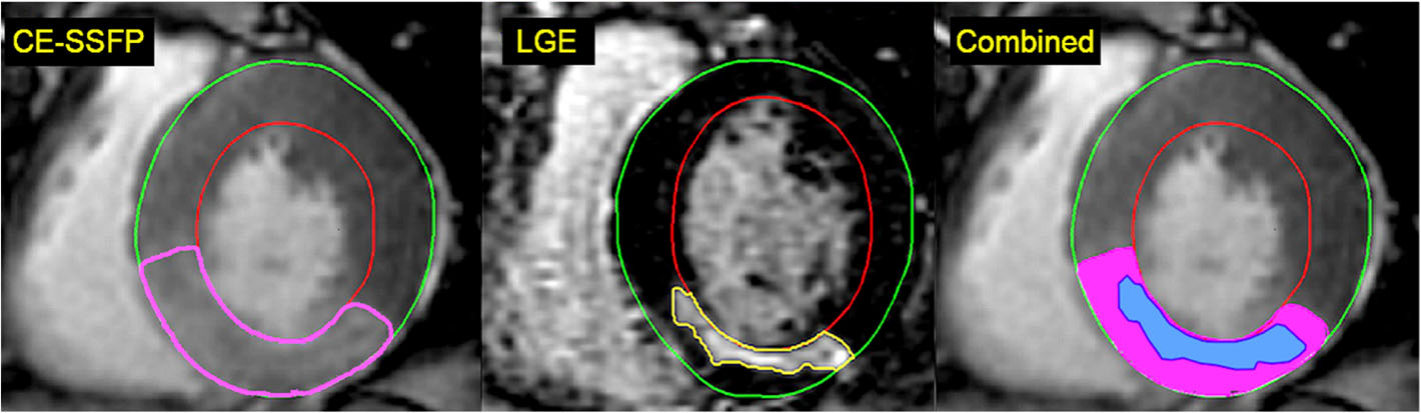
Example of MaR and myocardial salvage index using contrast-enhanced SSFP magnetic resonance imaging. Corresponding left ventricular short axis views from a patient with an inferior STEMI caused by a right coronary artery occlusion. The epicardium is delineated by a *green* border, and the endocardium by a *red* border. The myocardium at risk (MaR) in the inferior segment is determined by area of early gadolinium enhancement on SSFP (delineated by a *pink* border in the *panel to the left*). The size of infarction in the inferior segment is determined by the area of late gadolinium enhancement delineated by a *yellow* border in the *middle panel*. Automatic computer-aided superimposition of the infarcted area and the MaR area can be used to calculate the myocardial salvage index (as a ratio of the two areas, 1-scar area/MaR), *panel to the right*. MaR = myocardium at risk; CE-SSFP = contrast-enhanced steady-state free precession; STEMI = ST-elevation myocardial infarction; LGE = late gadolinium enhancement
